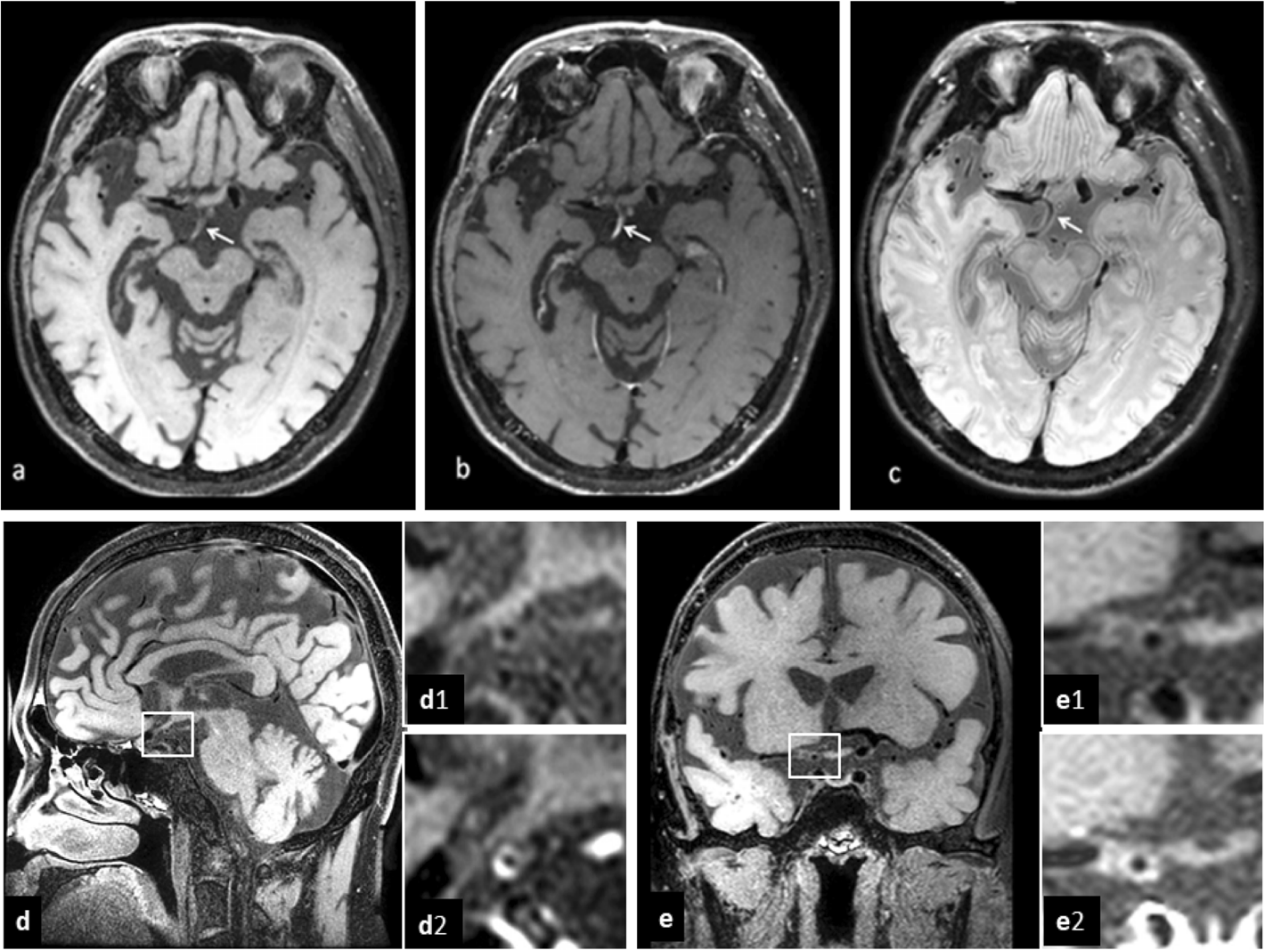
Brain high-resolution magnetic resonance imaging (HR-MRI). a-c: Mild T1 hyperintensity within the right posterior communicating artery wall in T1 pre-contrast HR-MRI, incomplete enhancement in T1 post-contrast HR-MRI and narrow lumen in PD-weighted HR-MRI. d-e: The right posterior communicating artery(d) and the right middle cerebral artery(e), concentric thickening of vascular wall in HR-MRI T1 sequence(d1, e1) and strong and concentric wall enhancement in T1 post-contrast HR-MRI(d2, e2)
